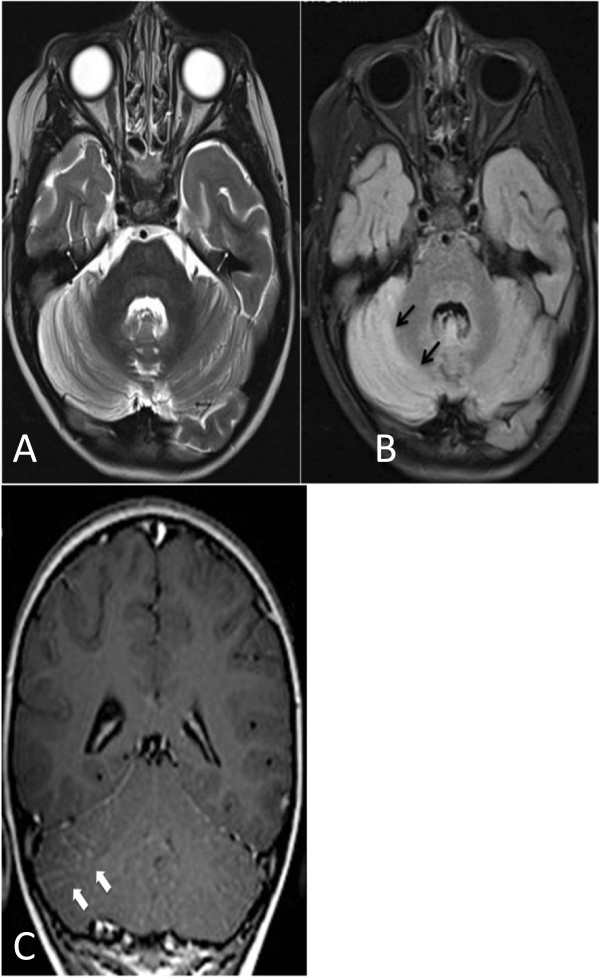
MRI images. A: axial T2-weighted image; B: axial FLAIR sequence. Diffuse high signal intensity of both cerebellar hemispheres with prevalence on the right lobe (black arrows). C: axial T1-weighted image after administration of paramagnetic contrast: pial disomogeneus contrast-enhancement of both cerebellar hemispheres, more evident on the right lobe (white arrows).
